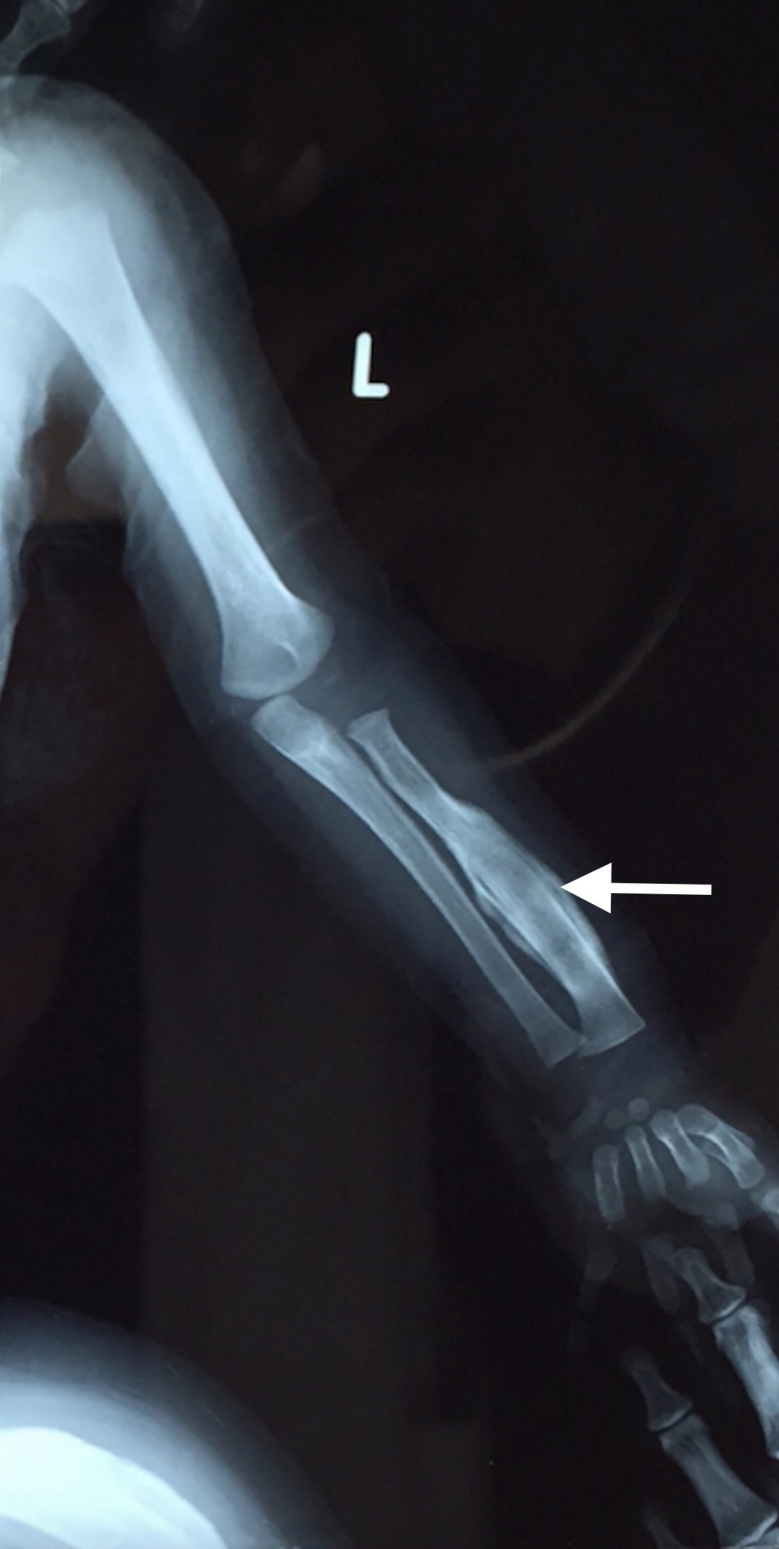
Anteroposterior (AP) view of left upper limb Radiograph shows complete encasement of radius by thick periosteal new bone with resultant cortical widening (arrow). Relative sparing of epiphysis and metaphysis is noted.
